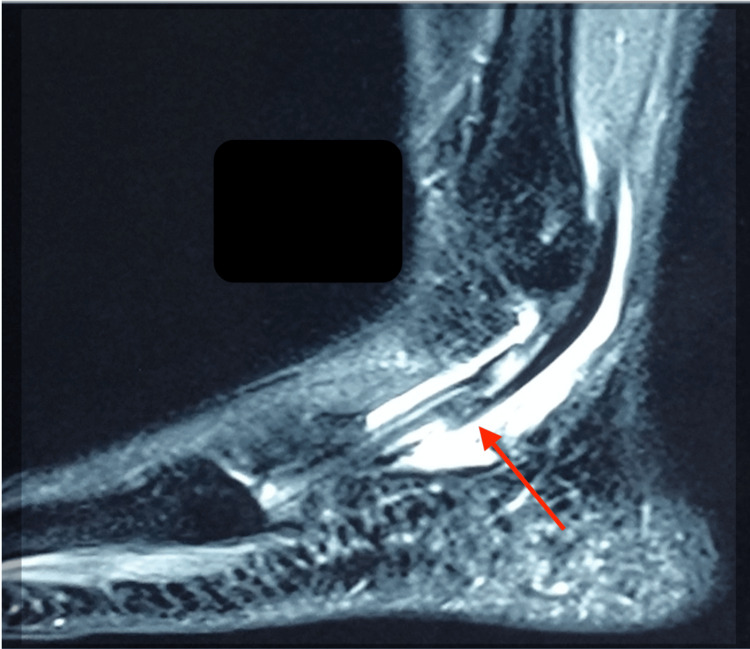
MRI sagittal view of the patient’s right ankle showing the longitudinal split (red arrow) in the peroneal brevis with fluid in the sheath.
